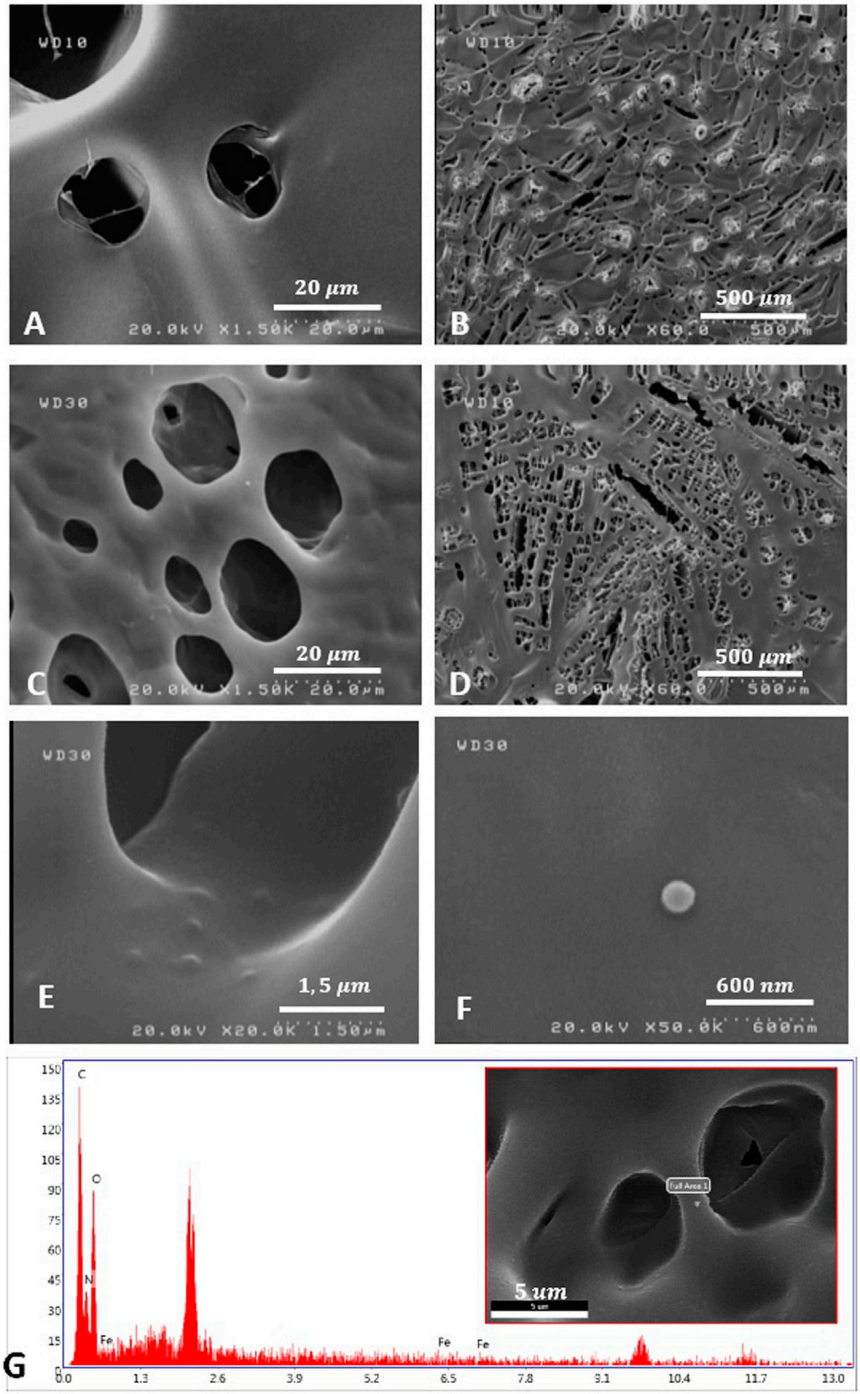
The morphology observation of SFH (A,B), and SFH/MNP1 (C–F) using FESEM. (G) Material characterization of SFH/MNP1 using Energy Dispersive X-Ray Analysis (EDAX) in the selected area.
